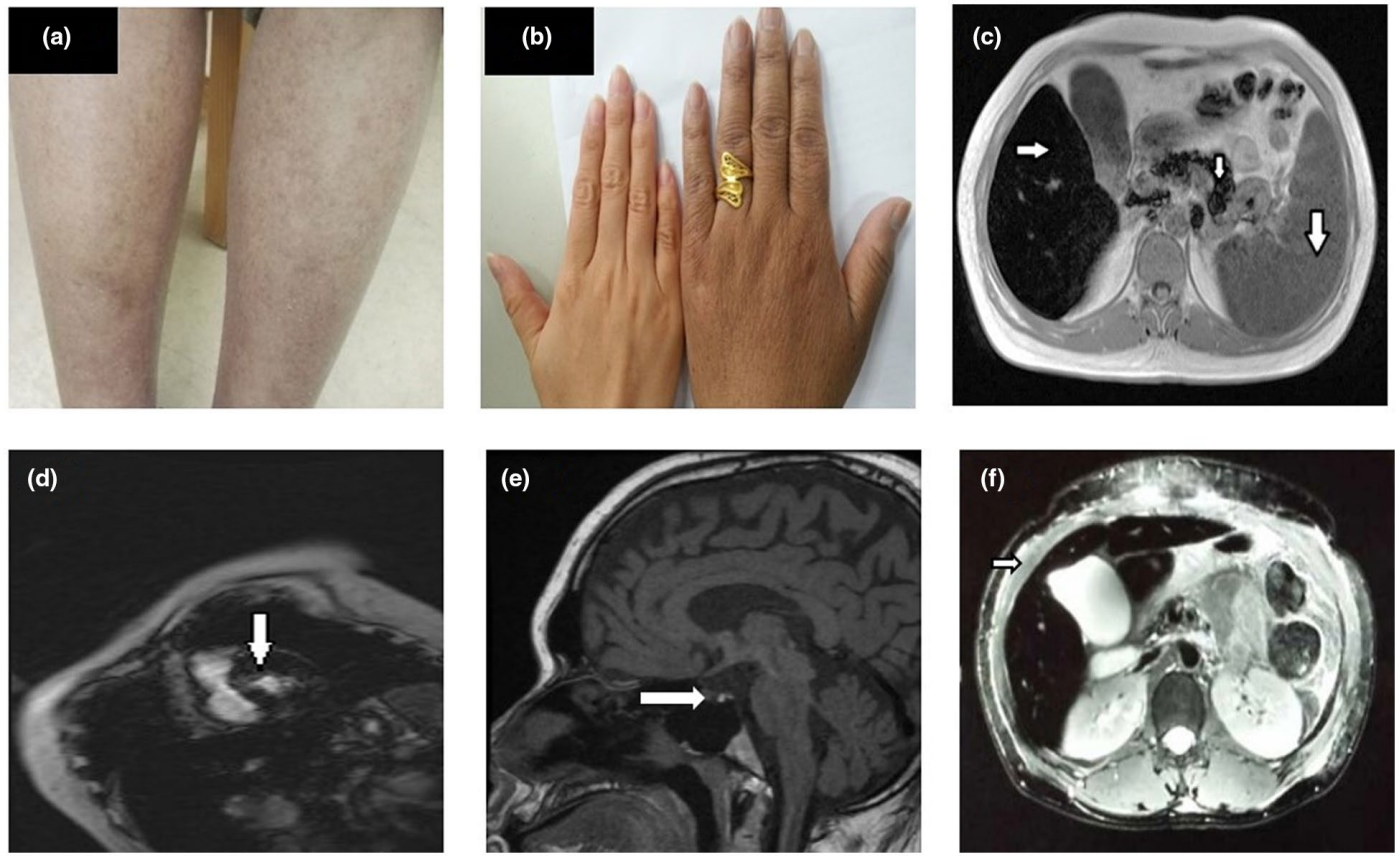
Focal skin pigmentation on limbs and MRI scan for two cases. (a) The slight pigmentation of the anterior tibia in 1‐III‐1. (b) The pigmentation of the left hand of 2‐II‐1. (c–e) The abnormal deposition of iron in the liver, myocardium, and pituitary, respectively, by MRI in 1‐III‐1. (f) The abnormal deposition of iron in the liver in 2‐II‐1
